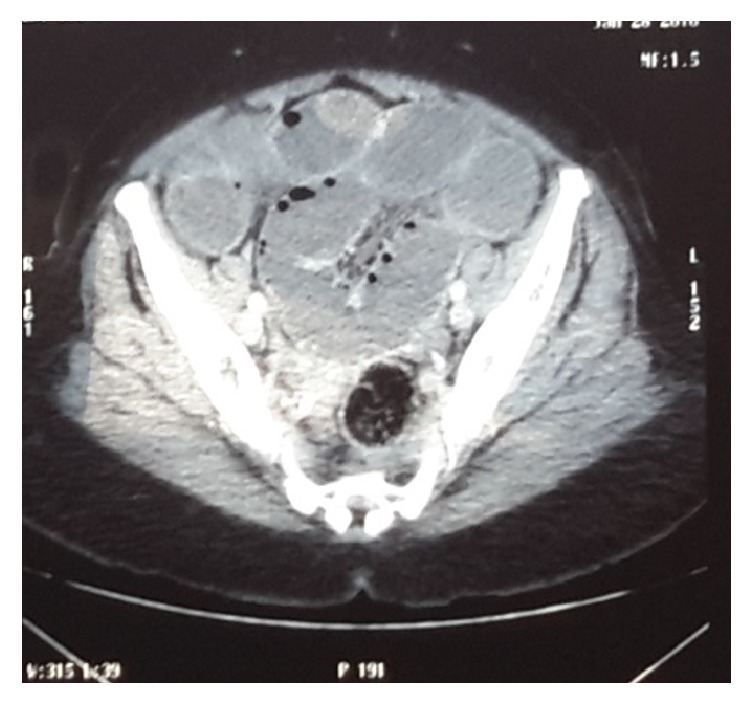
Axial view of computed tomography (CT) of the abdomen showing grossly dilated, thick-walled small bowel loops up to the region of the pelvis.
